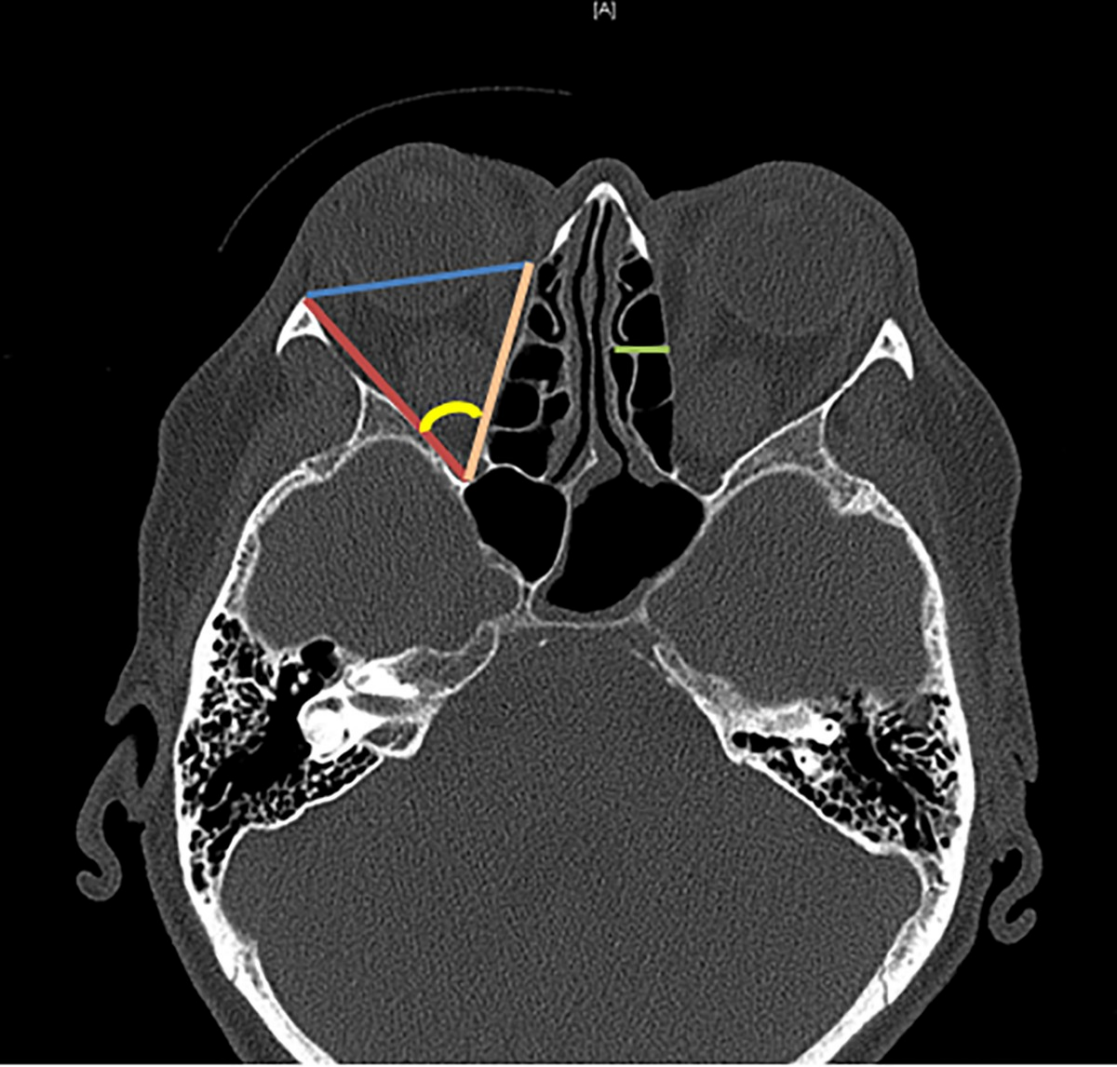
Pre- and postoperative computed tomography images were analyzed. The orbital area was measured as a triangle comprised of lateral (red) and medial orbital wall (orange), as well as orbital width (blue). Furthermore, the conus angle (yellow) and the depth of the ethmoid cavity were measured. Postoperative scans at the same level were used to measure length of medial and orbital defect and depth of medial tissue prolapse.
